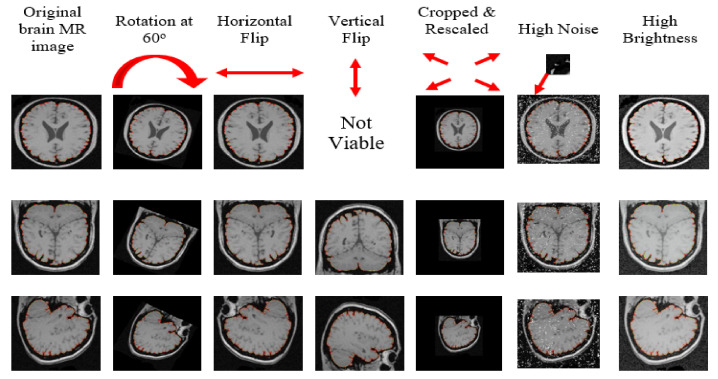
Augmentation of brain MR images reserved for training.
